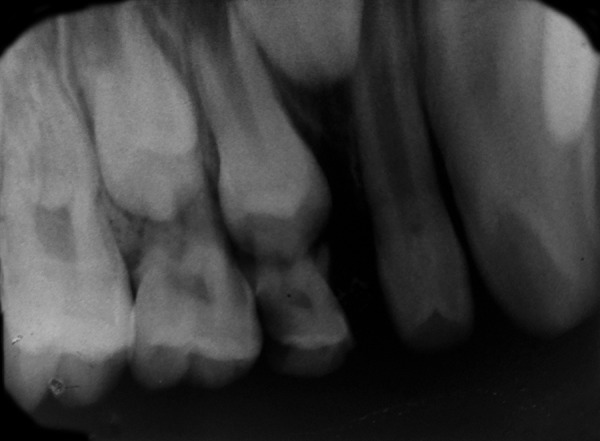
Intraoral periapical radiograph wrt 64 showing physiologic resorption
